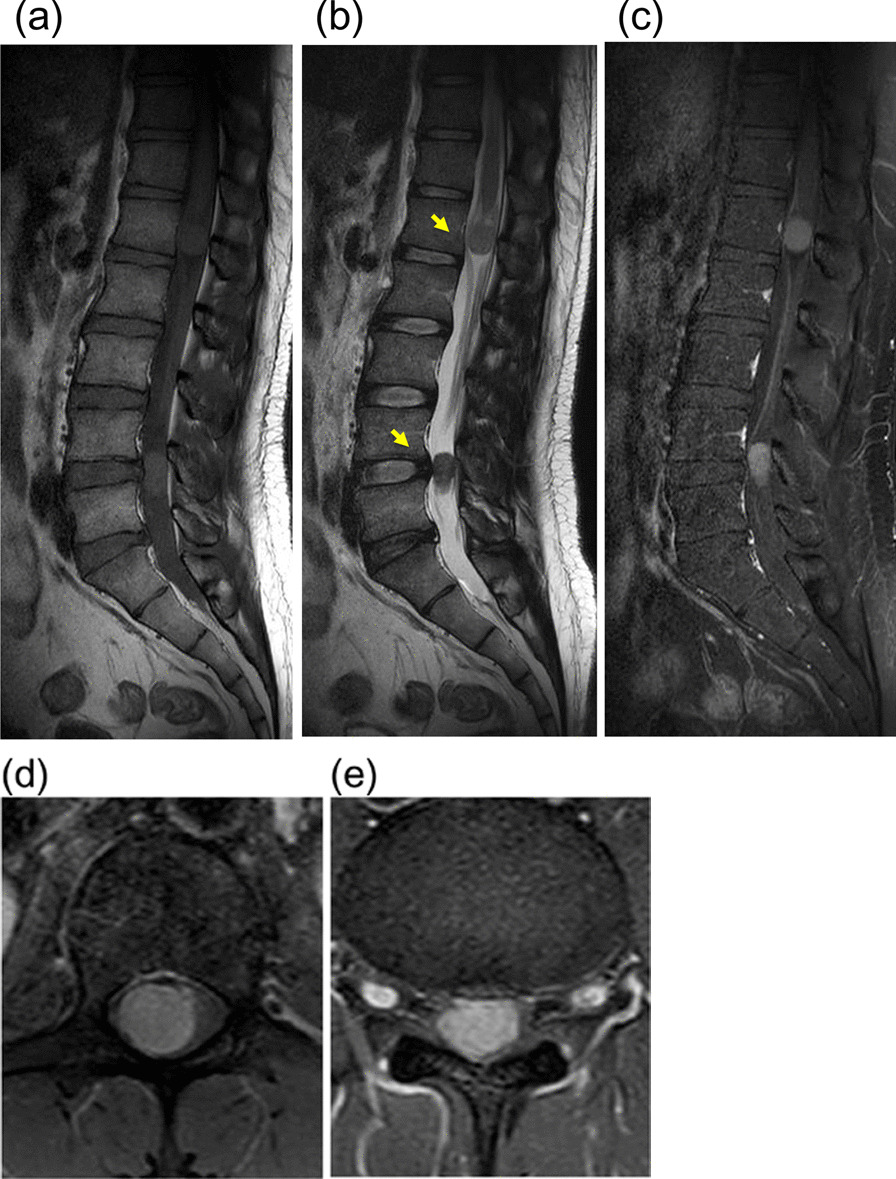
Lumbar MRI showing two intradural masses (arrows) without “dural tail signs” at the L1 and L4/L5 levels on the T1-weighted image (a) and T2-weighted image (b). Gadolinium-enhanced T1-weighted MRI images showed the masses with homogeneous enhancement on the sagittal image (c) and the axial images at the L1 (d) and at L4/5 (e) levels
